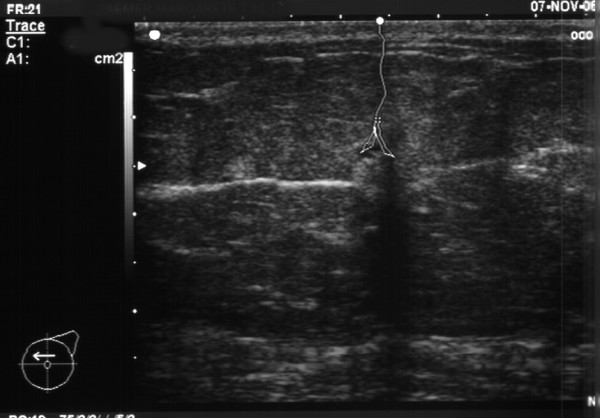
Breast-ultrasound for routine follow-up of a patient (no.17, 16 years after primary breast cancer) showed a small hypoechogeneity within the previous index quadrant.
